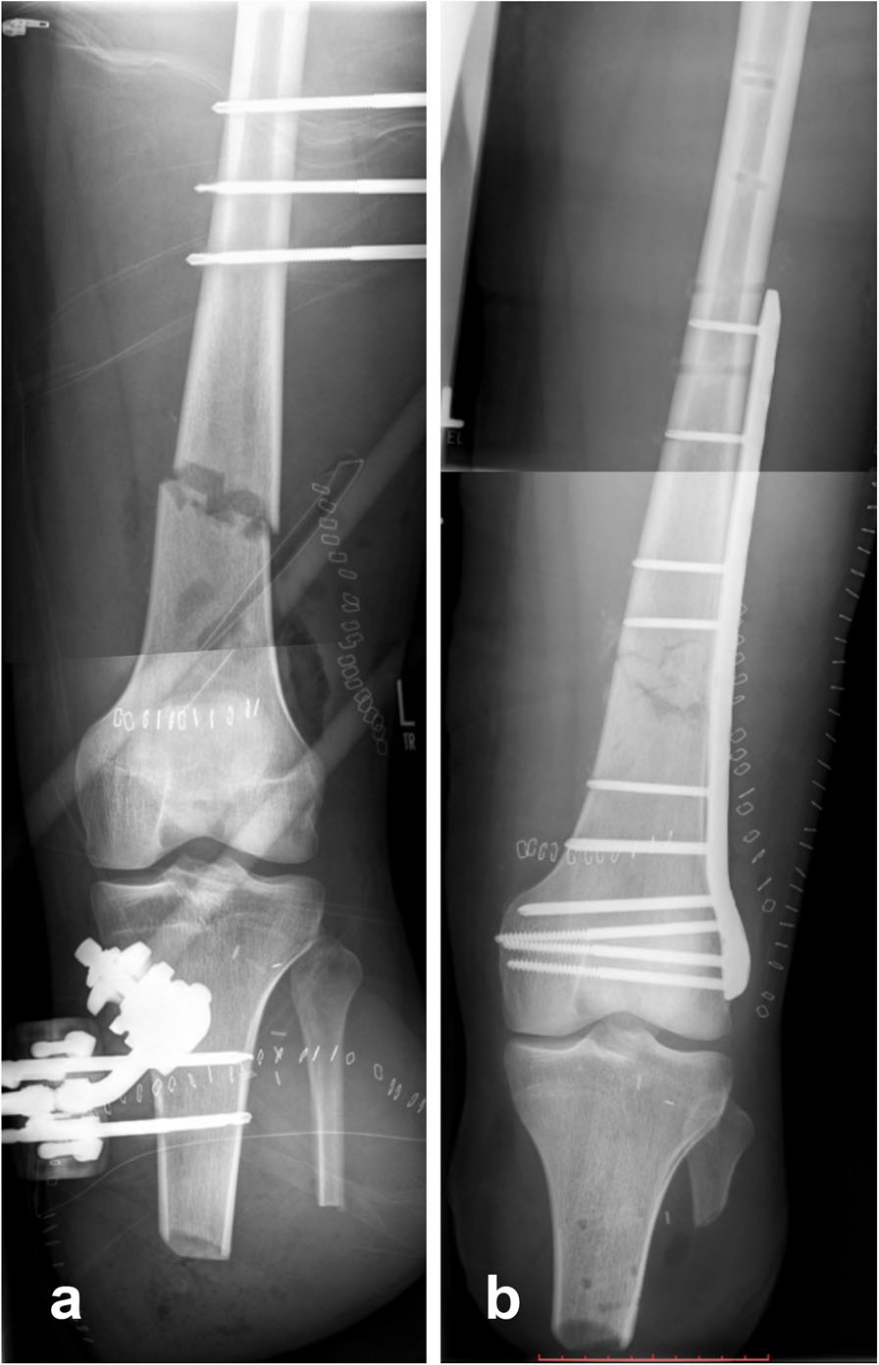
Anterior posterior X-ray of the left lower extremity. a After transport of the patient from Spain to our hospital, with lower leg stump and external fixator, after traumatic amputation of the lower leg and open, multi-fragmentary, distal femoral fracture (day 1). b After definitive surgery, with a closed, infection-free amputation stump and after open reduction and stabilization of the multi-fragment distal femur fracture with an angular stable plate osteosynthesis (day 20)
